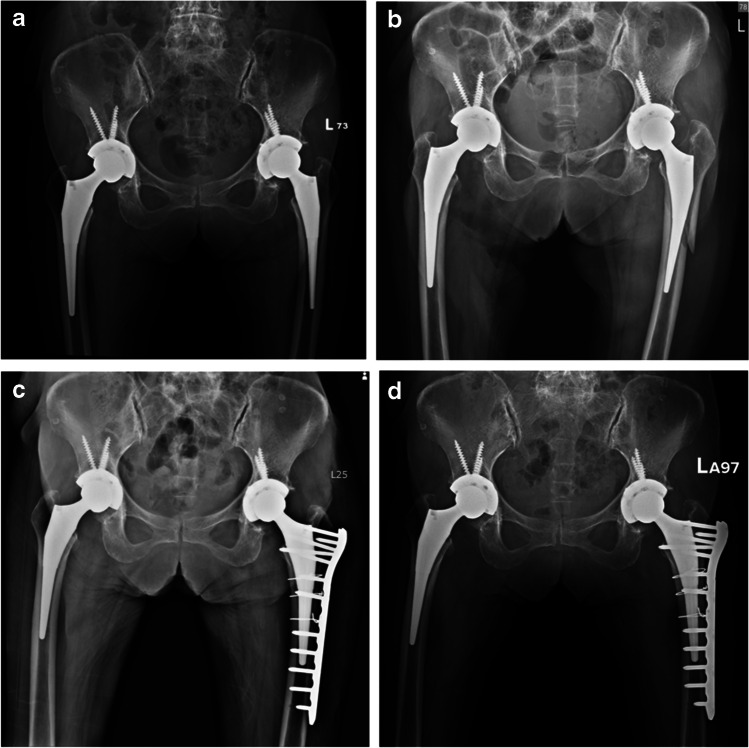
The series of events and treatments for an 83-year-old female patient who underwent a left total hip arthroplasty and subsequently experienced complications: a Initial: The initial x-ray image shows the patient's hip joint after receiving a left total hip arthroplasty. b Complication: The patient suffered an accidental fall, which resulted in a post left total hip arthroplasty complication—a peri-prosthetic fracture, classified as VTB2. A fracture around the stem of the implant with a loose prosthesis, requiring revision surgery. c Revision surgery: To address the complication, the patient underwent a revision surgery. A short taper stem was used to replace the damaged prosthesis, and open reduction internal fixation with a VA condylar locking compression plate was performed to stabilize the fracture. d Follow-up: After 12 months of follow-up, the patient showed no signs of stem subsidence or implant failure. She was able to return to her daily activities without any significant issues
